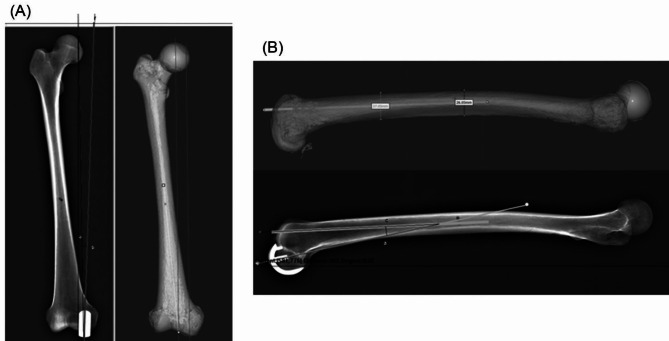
Postoperative coronal and sagittal alignment of the femoral prostheses evaluated using X-ray radiography and measured using e-Ruler software. (A) anteroposterior-view radiographs to assess the coronal alignment, which was defined as the angle between the alignment of prosthesis (line a) and the femoral mechanical axis (line b). The left panel shows the radiological outcomes and the right panel shows femoral prostheses. (B) lateral view-radiographs to assess the sagittal alignment, which was defined as the angle between the alignment of prosthesis (a) and the anatomical axis (c). The top panel shows femoral prostheses and the bottom panel shows radiological outcomes
